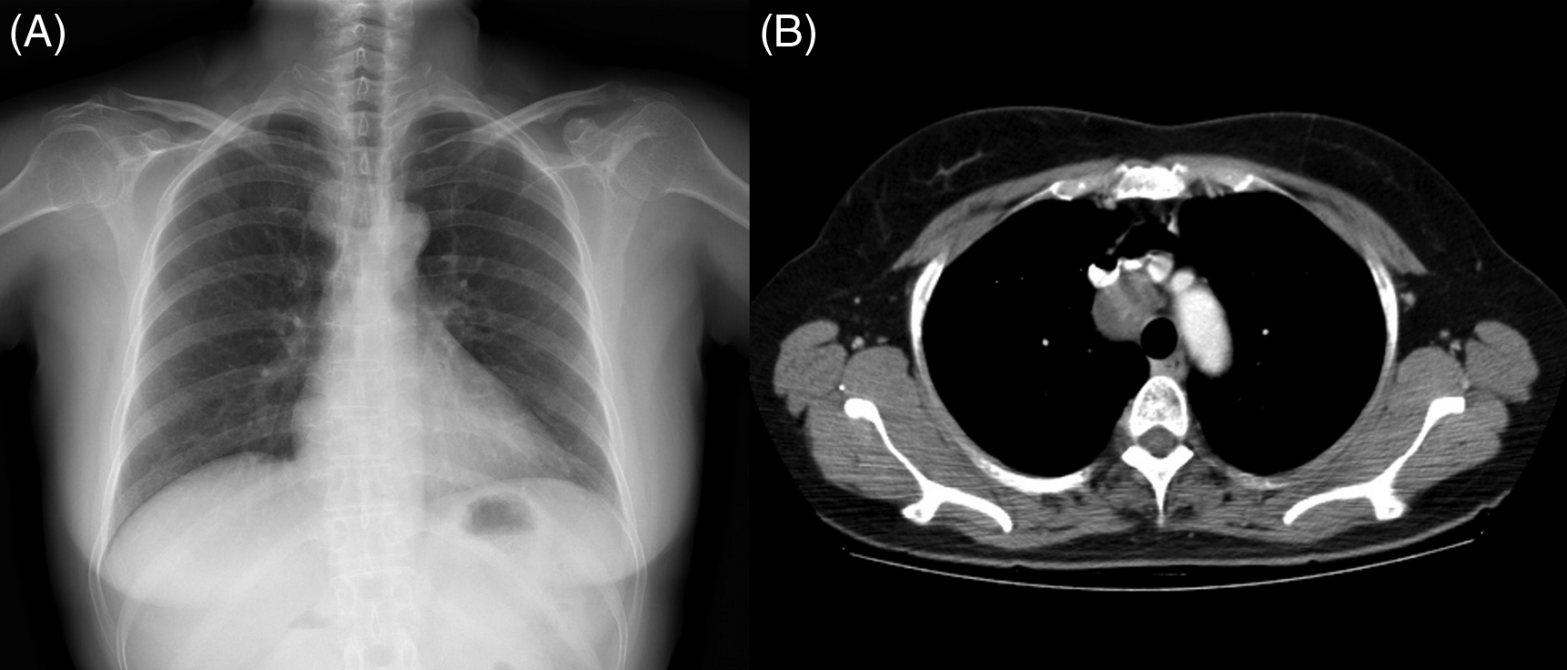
Chest imaging. (A) Chest X‐ray shows right mediastinal enlargement. (B) Computed tomography of chest reveals a soft tissue density in the right paratracheal area.
